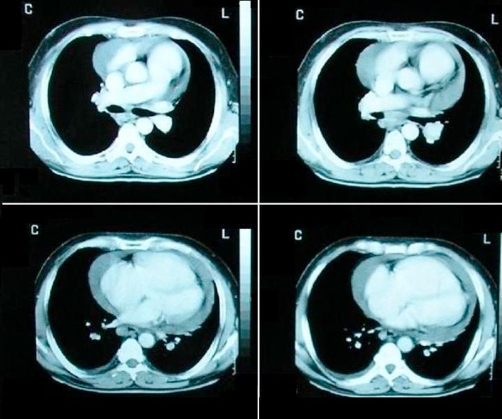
Pericardial fluid (CT of the thorax).
